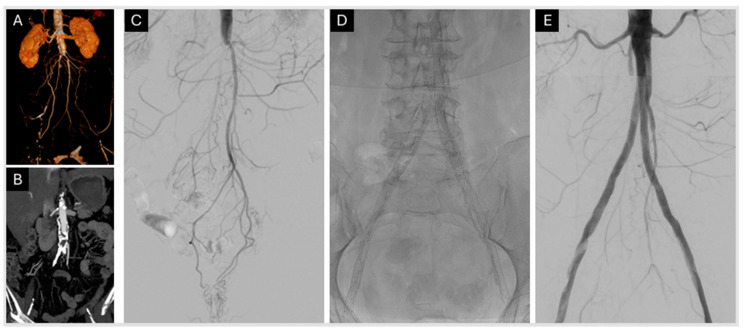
Case example using the kissing stent technique. (A) 3D reconstruction based on pre-interventional CT imaging; (B) pre-interventional CT demonstrating aortoiliac occlusive disease; (C) diagnostic angiography before intervention; (D) fluoroscopic image after reconstruction using the kissing technique; (E) final angiography showing the post-interventional result. CT, computed tomography.
